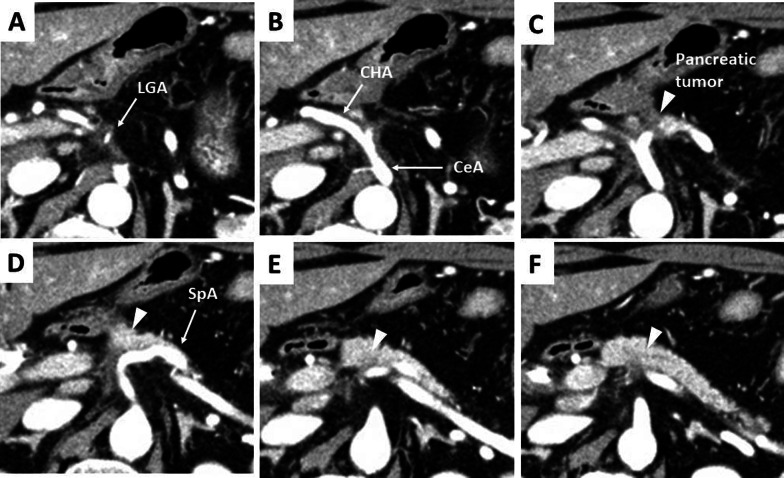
A computed tomography (CT) findings after five cycles of chemotherapy. A, B The soft tissue mass surrounding the left gastric artery (LGA) and CeA slightly remained. C–F The pancreatic tumor (arrowhead) and lymph node shrunk showing improvement in the deformity of the splenic artery (SpA) and involvement of the celiac artery (CeA) and common hepatic artery (CHA). E, F The dilatation of the main pancreatic duct was disappeared
